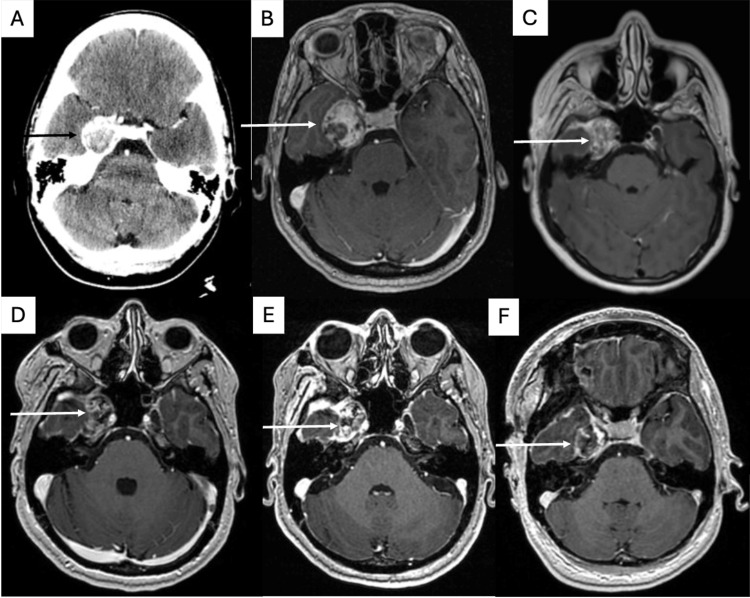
Right cavernous sinus malignant melanotic nerve sheath tumor (Case 1). Axial skull base images, with arrows indicating the tumor, including (A) CT and (B) preoperative MRI demonstrating a T1-hyperintense mass centered in the middle cranial fossa. Postoperative MRIs demonstrate (C) residual tumor one day after surgery, (D) tumor extent immediately prior to SRS, (E) the irradiated tumor at the first follow-up, two months after SRS, and (F) stable residual disease 3.5 years following SRS. SRS: stereotactic radiosurgery.
